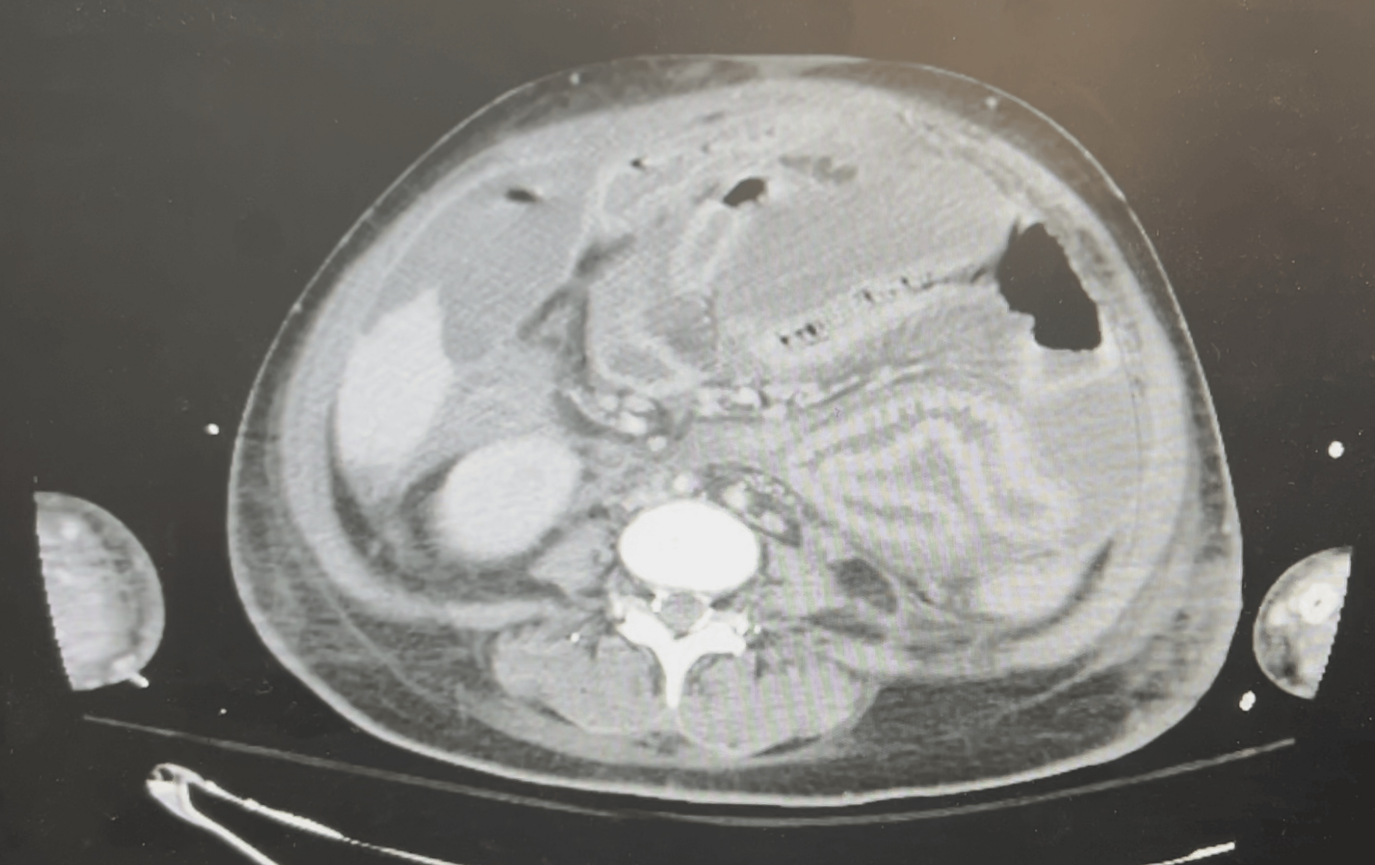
Abdominal CT scan demonstrating large intra-abdominal fluid collections, likely consistent with uroperitoneum, indicative of uroperitonitis
